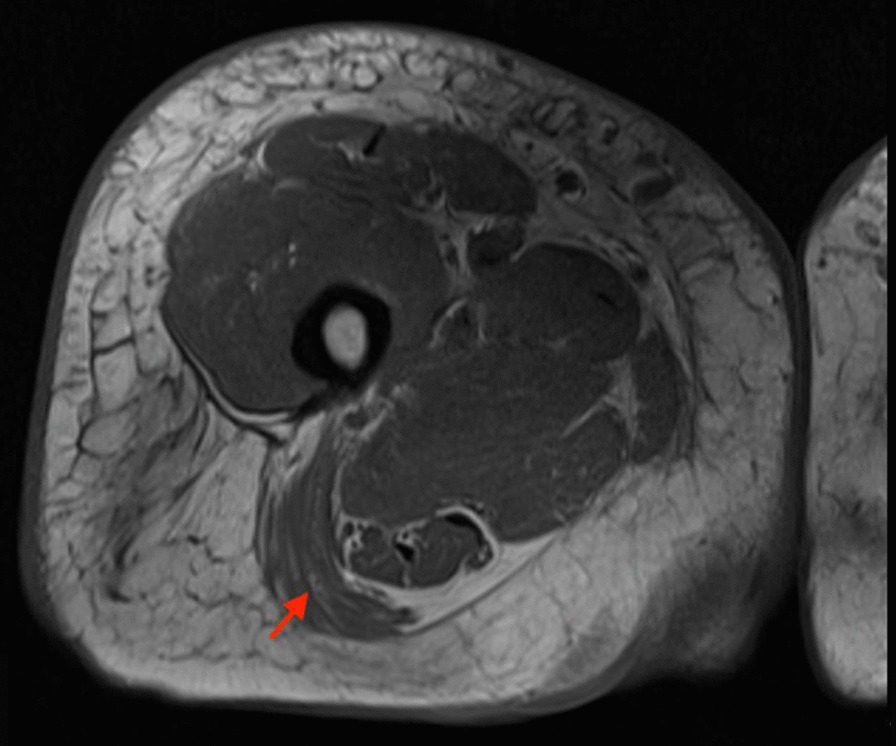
MRI right thigh with arrow showing perimysial edema surrounding the biceps femoris muscles
